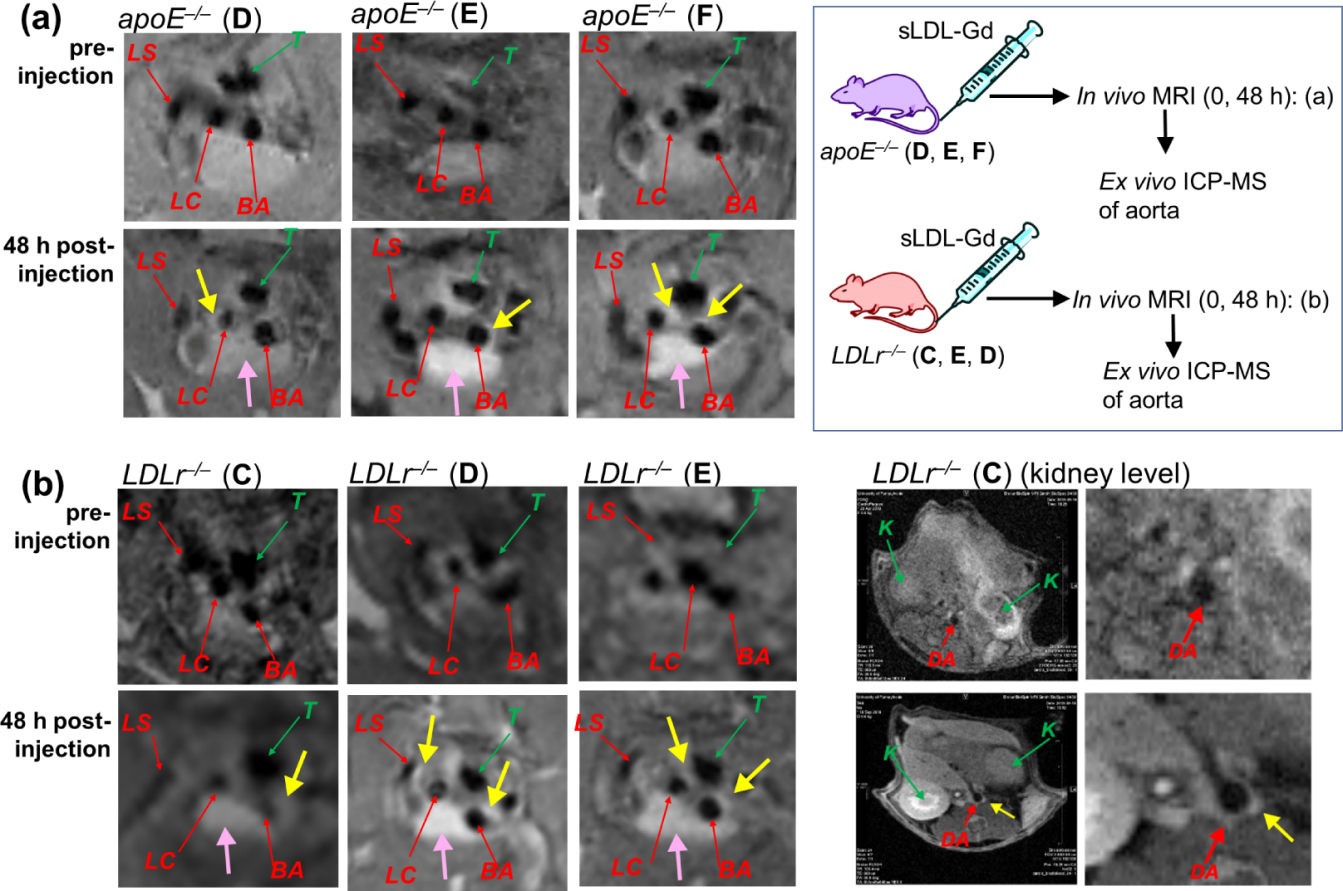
In vivo* MR images of the aortic arch
